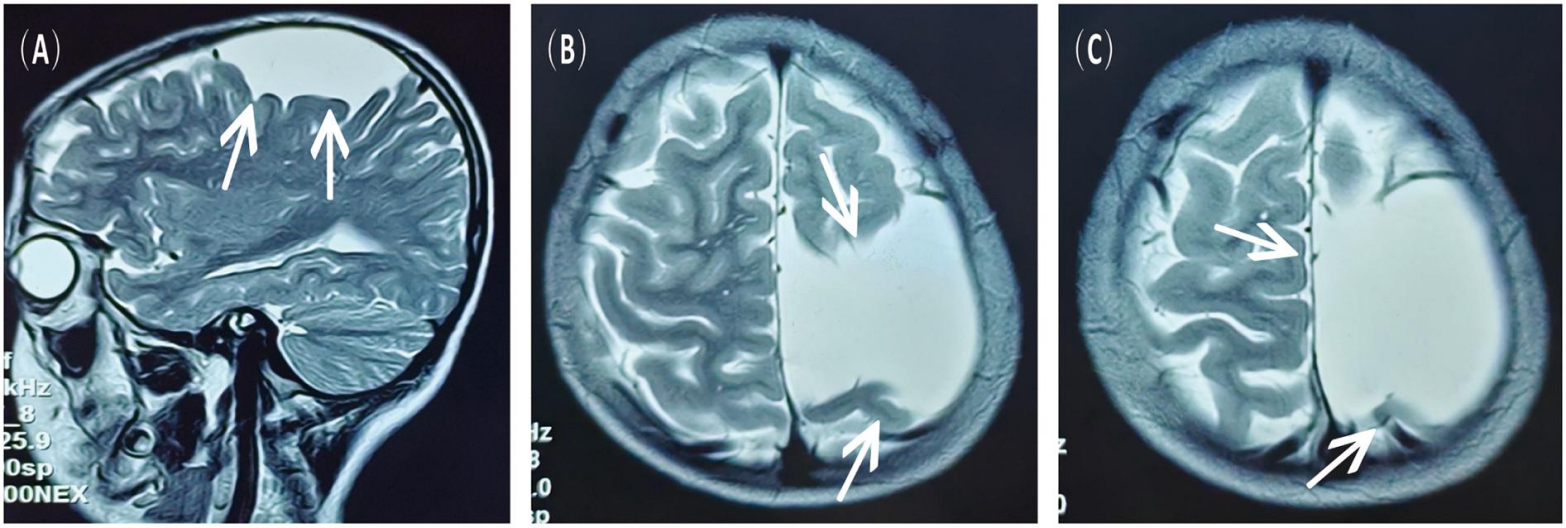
MRI scans obtained 3 years prior to the current admission, showing the left fronto-temporo-parietal arachnoid cyst. (A) Sagittal view, T2-weighted sequence. (B,C) Axial view, T2-weighted sequence.
